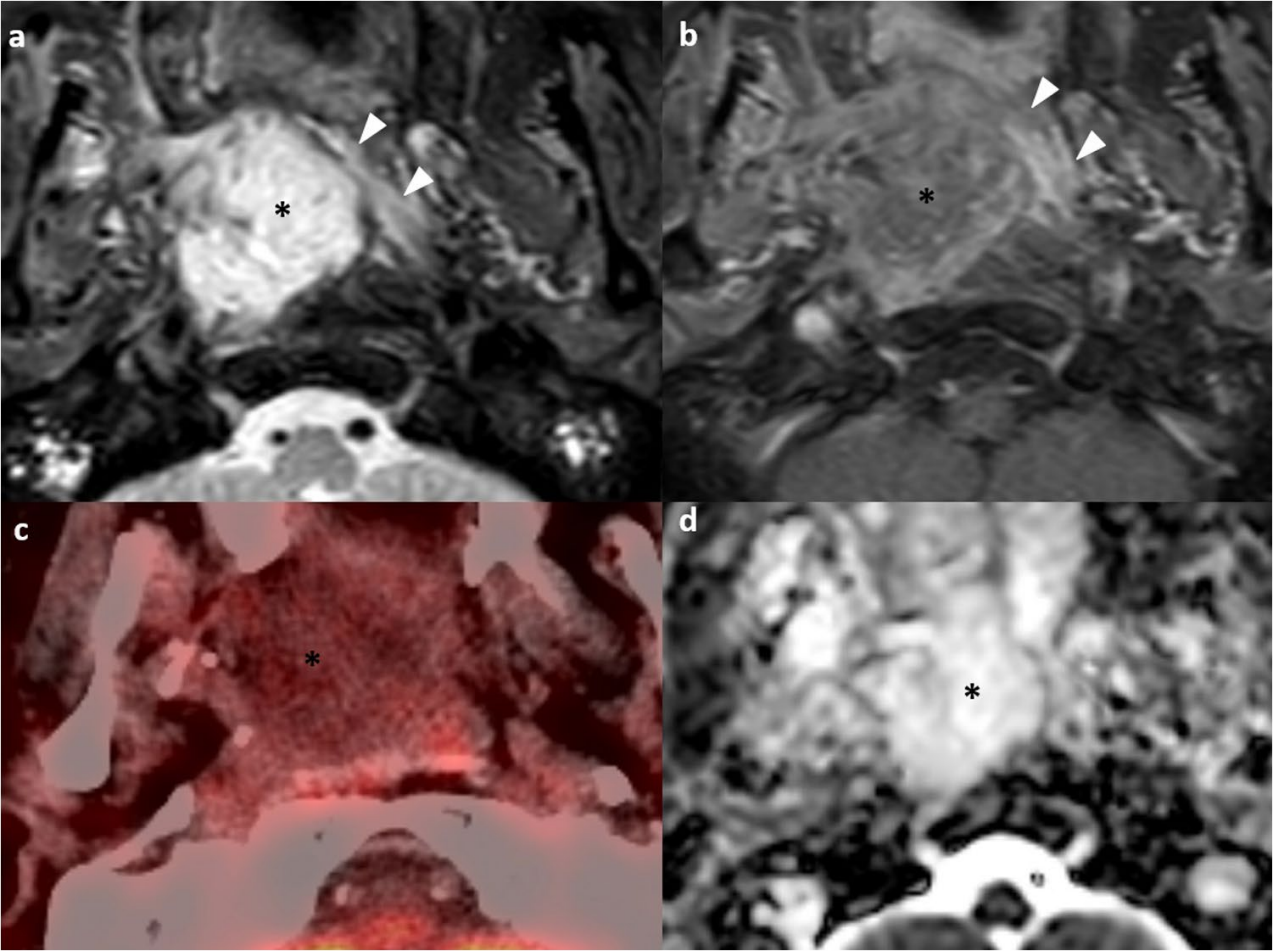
Same patient as Fig. 6. Normal expected early post-operative appearance of open nasopharyngectomy in a 72-year-old man with recurrent NPC (clinical restaging T2N0M0). He had open right nasopharyngectomy via maxillary swing approach with vastus lateralis flap followed by post-operative stereotactic radiotherapy. A follow-up MRI was performed at 2-month after the operation. Axial T2-weighted image with fat-suppression shows the flap at the right nasopharynx appearing diffusely swollen with markedly hyperintense signal (asterisk) with perifocal soft tissue edema (arrowheads) (a). Axial T1-weighted post-contrast image with fat suppression shows the flap with heterogenous enhancement (asterisk) and perifocal soft tissue enhancement in the operative bed (arrowheads) (b). The imaging appearance of the flap was confused with residual tumor and additional FDG-PET/CT as well as follow-up MRI with DWI were performed. Axial fusion FDG-PET/CT shows the flap being diffusely eumetabolic (asterisk) (c). Conventional sequences on follow-up MRI after a further 2 months showed no interval change in size and signal characteristics of the flap (not shown). ADC map shows that there is diffuse hyperintense signal within the flap suggestive of facilitated diffusion (asterisk) (d). No residual or recurrent tumor was evident
